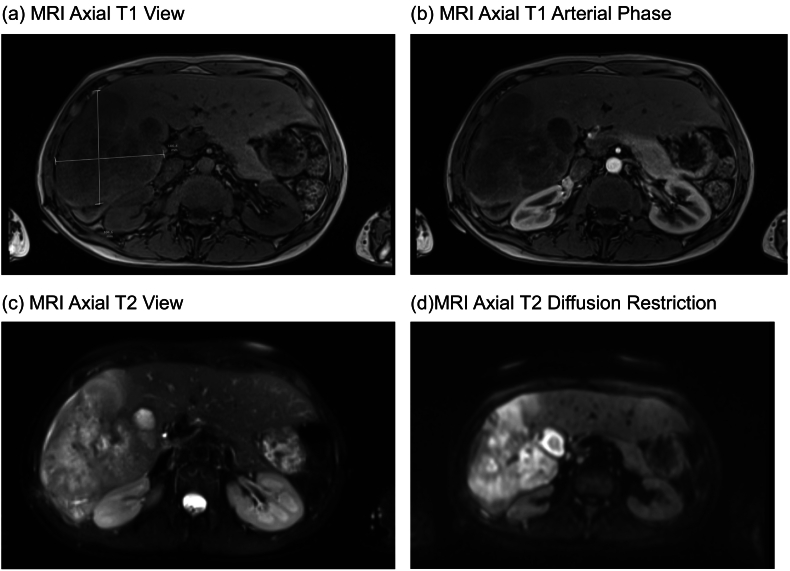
MRI liver – axial T1 view, T1 arterial phase, T2 View, & T2 diffusion restriction.
